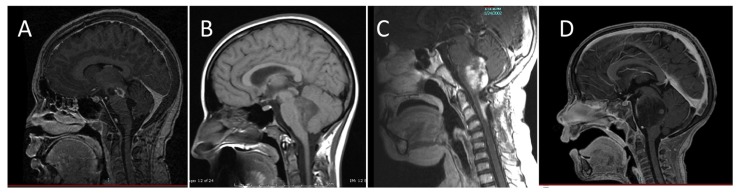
Classification of brainstem gliomas by MRI appearance. (A) Focal brainstem lesion on T1-weighted post-contrast sagittal image. (B) Dorsal exophytic brainstem lesion on sagittal non-contrast MRI. (C) Cervicomedullary lesion on T1-weighted post-contrast sagittal image. (D) Diffuse intrinsic pontine glioma lesion on T1-weighted post-contrast sagittal image.
